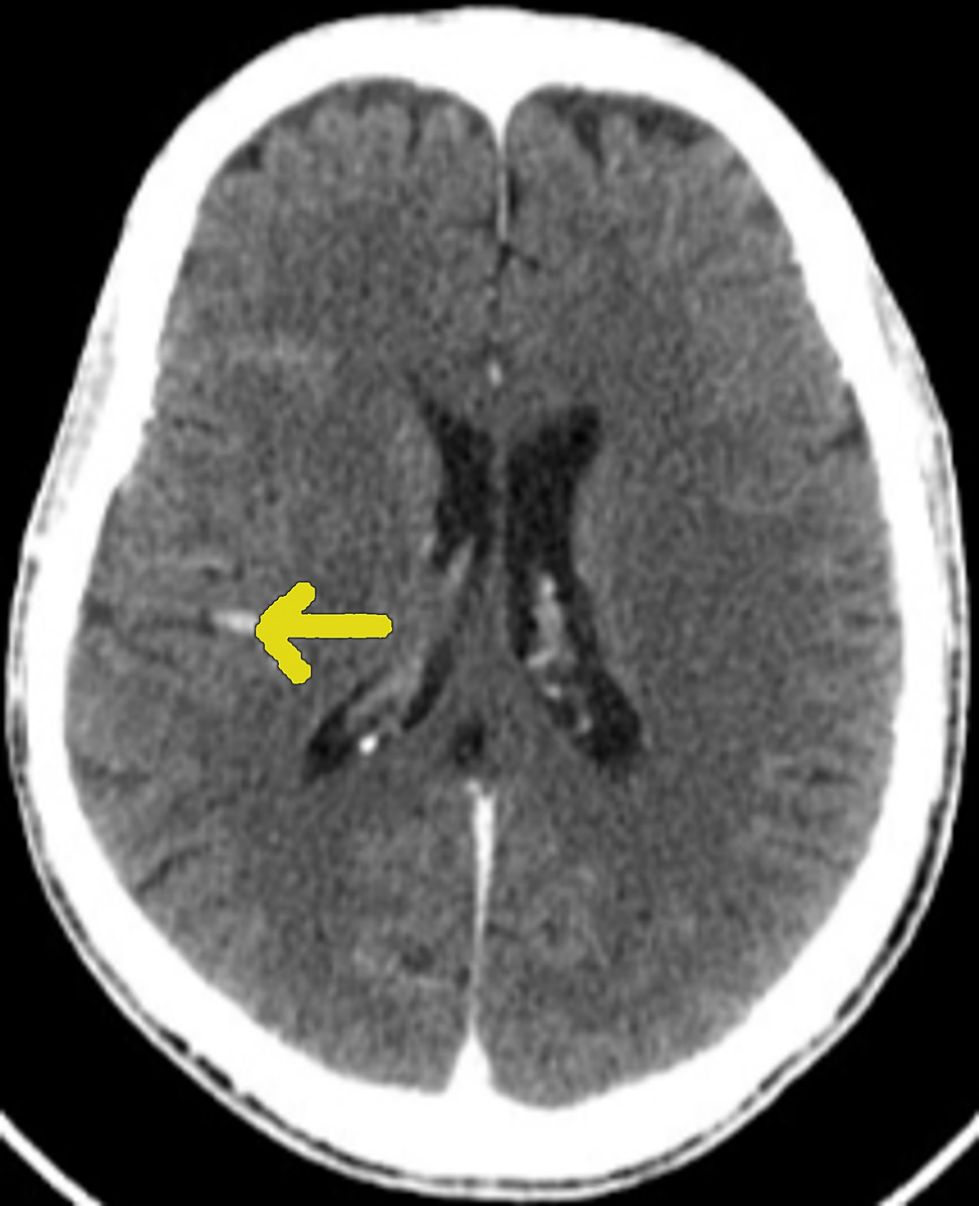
Cerebral CT image of an axial section showing a cerebral mycotic aneurysm.
